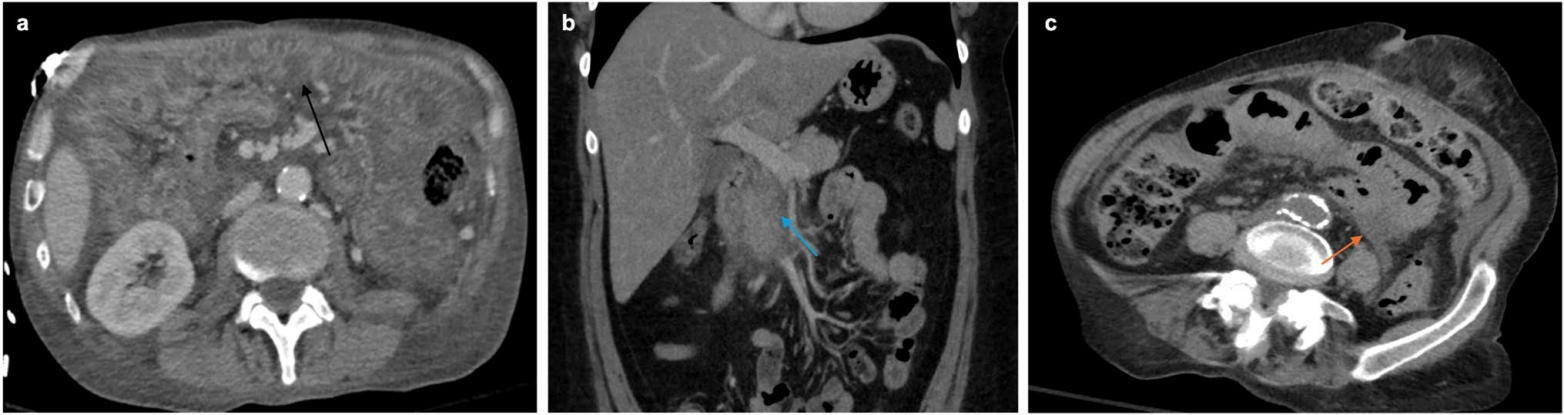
CT abdomen/pelvis axial view (a) demonstrates severe, diffuse treatment-associated colitis (black arrow), recognizable by nodular indentations of the lumen (aka thumbprinting), mucosal hyperemia, extensive fat stranding, wall thickening, and a pattern known as the accordion sign. Coronal CT abdomen (b) and axial CT (c) reveal pancreatitis (blue arrow) recognized by a markedly enlarged and irregular-appearing pancreas with extensive infiltration of peripancreatic fat in addition to peripancreatic fluid (orange arrow)
